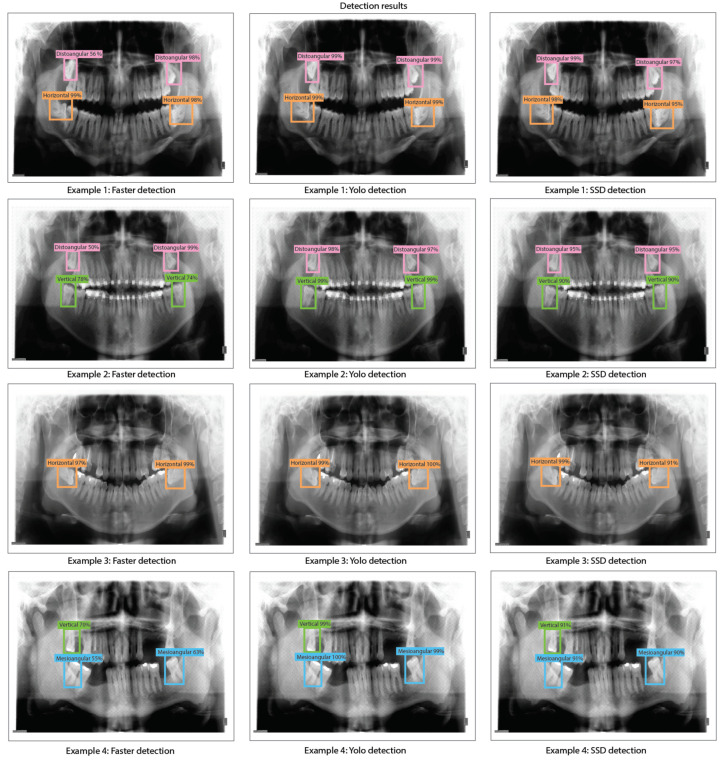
Third molar angle detection in dental panoramic X-rays with ResNet-101 as feature extractor for Faster R-CNN, YOLO V2, and SSD.
